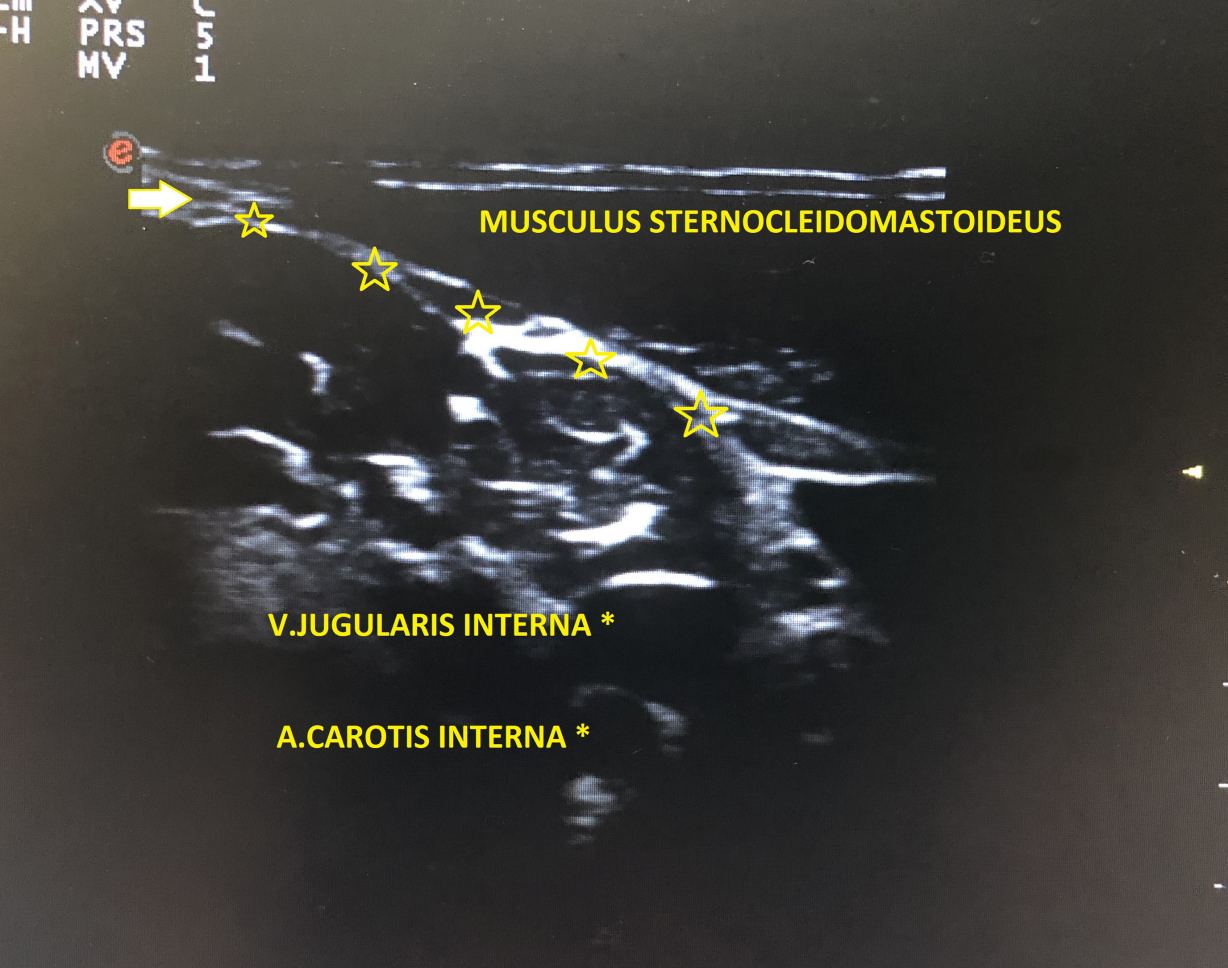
Anatomy under ultrasound. Yellow arrow shows block needle entry point. Yellow stars show local anaesthetic spread plane.
